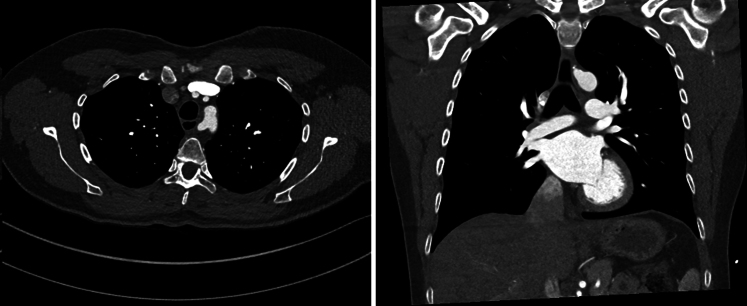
Contrast-enhanced computed tomography images demonstrating changes after robotic division of aberrant right subclavian artery. *Left*, axial view; *right*, coronal view.
